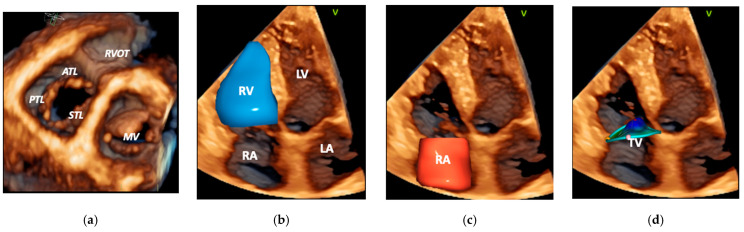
Utility of transthoracic 3DE to assess patients with tricuspid regurgitation. (a) Tricuspid valve functional anatomy. (b) Right ventricular volume and ejection fraction. (c) Right atrial size and function. (d) Tricuspid annulus geometry and valve tenting volume and height. Abbreviations: 3DE, three-dimensional echocardiography; ATL, anterior tricuspid leaflet; LA, left atrium; LV, left ventricle; MV, mitral valve; PTL, posterior tricuspid leaflet; RA, right atrium; RV, right ventricle; RVOT, right ventricular outflow tract; STL, septal tricuspid leaflet; TV, tricuspid valve.
